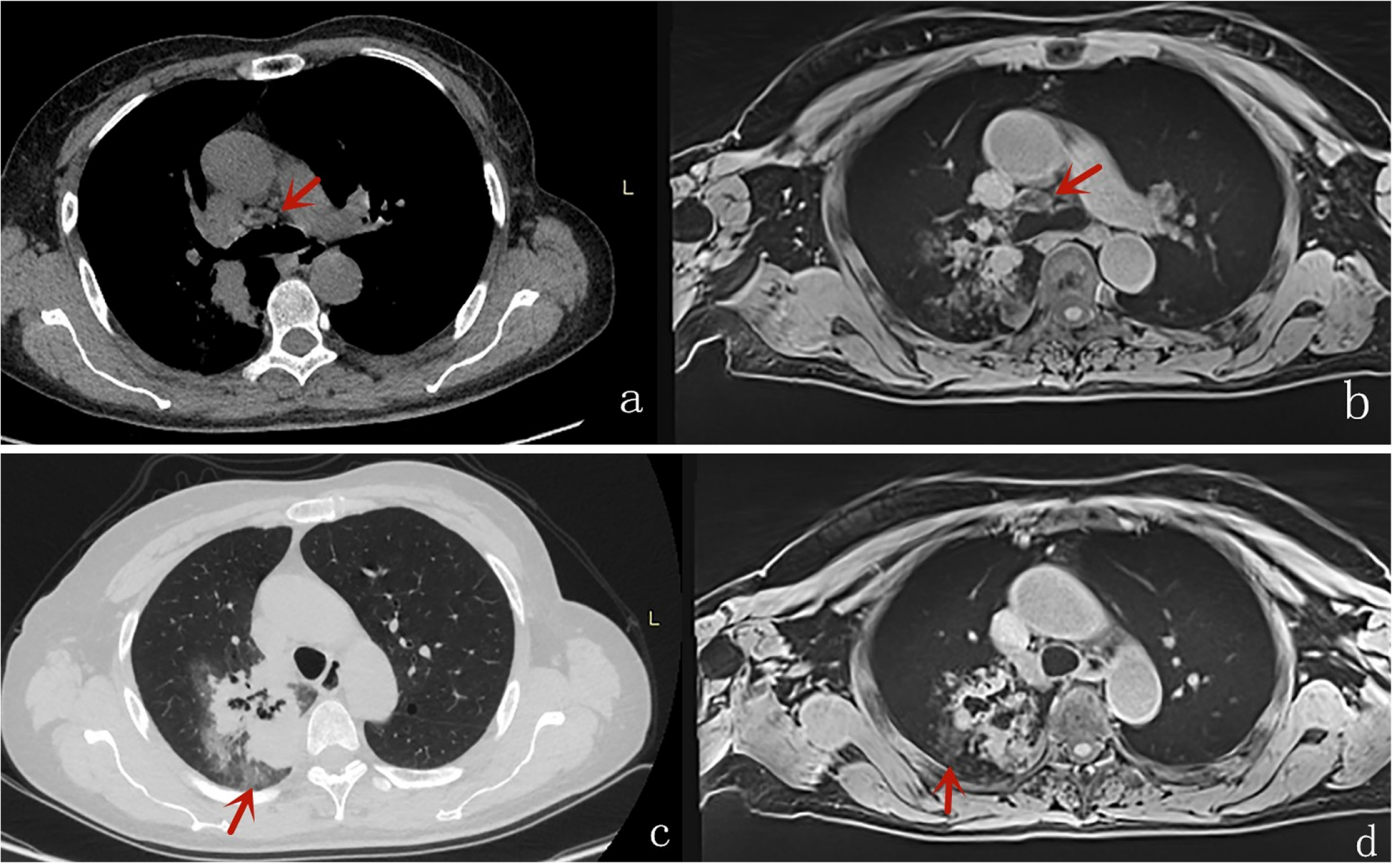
A female with acinar adenocarcinoma; axial CT (A) and r-VIBE (B) showed enlarged mediastinal lymph nodes (the red arrow). And sign of Halo was also visible in axial CT (C) and MR (D)
